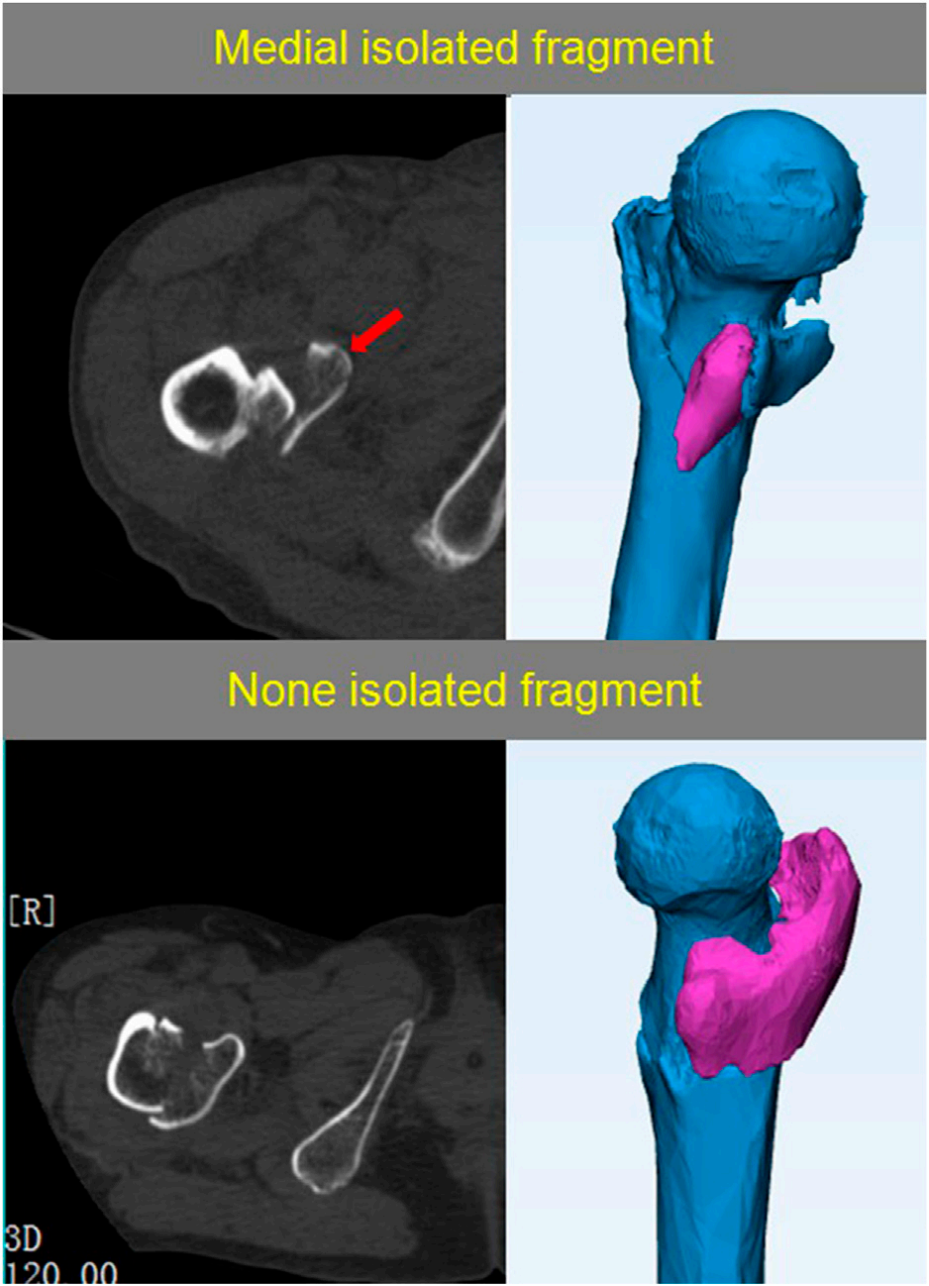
Using 2-d and 3-d CT images to determine medial isolated fragment. The red arrow shows medial isolated fragment.
